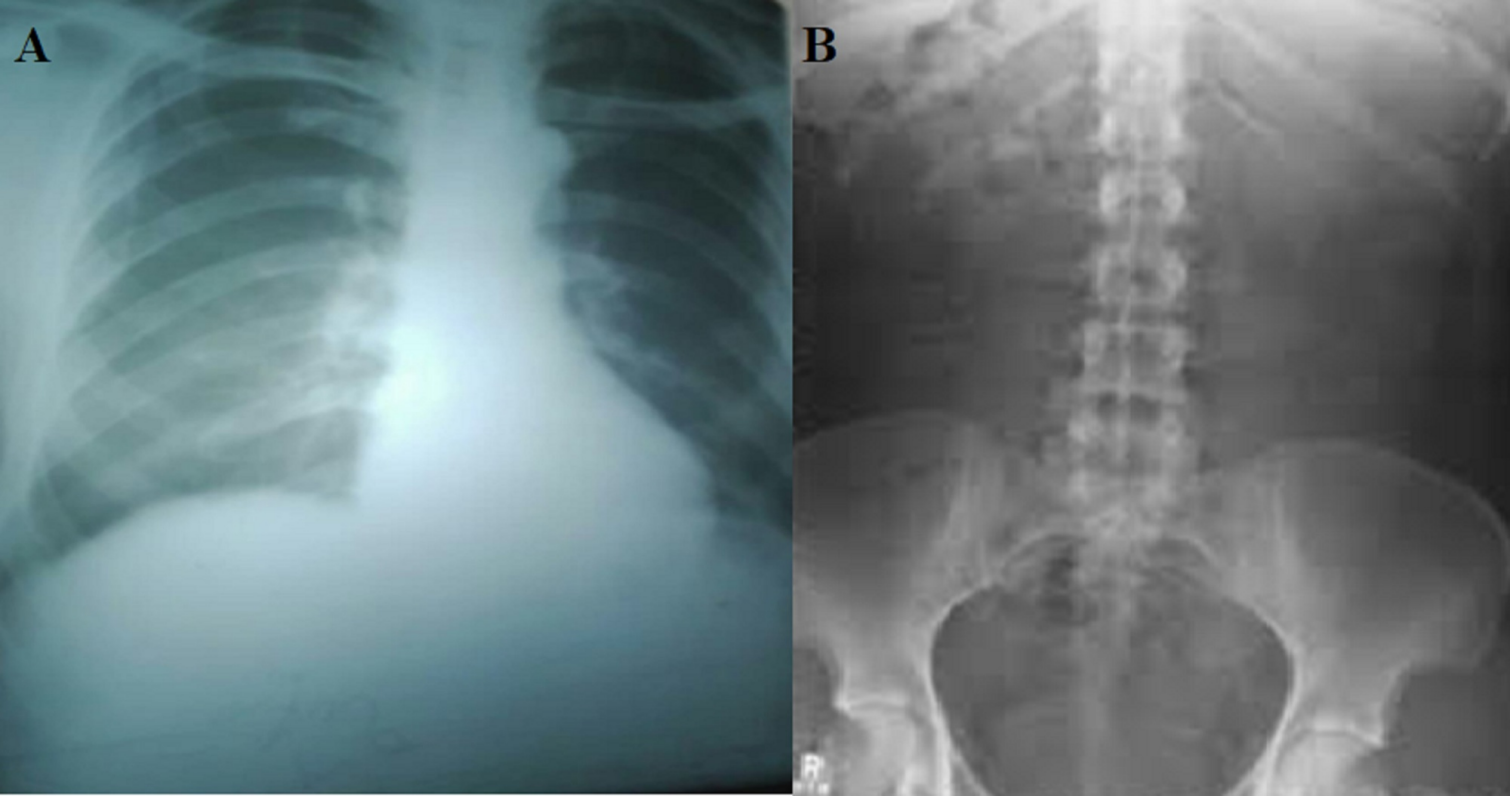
A) Chest X-ray showed no air under the diaphragm; B) Abdominal X-ray (erect) showed no gut distension
